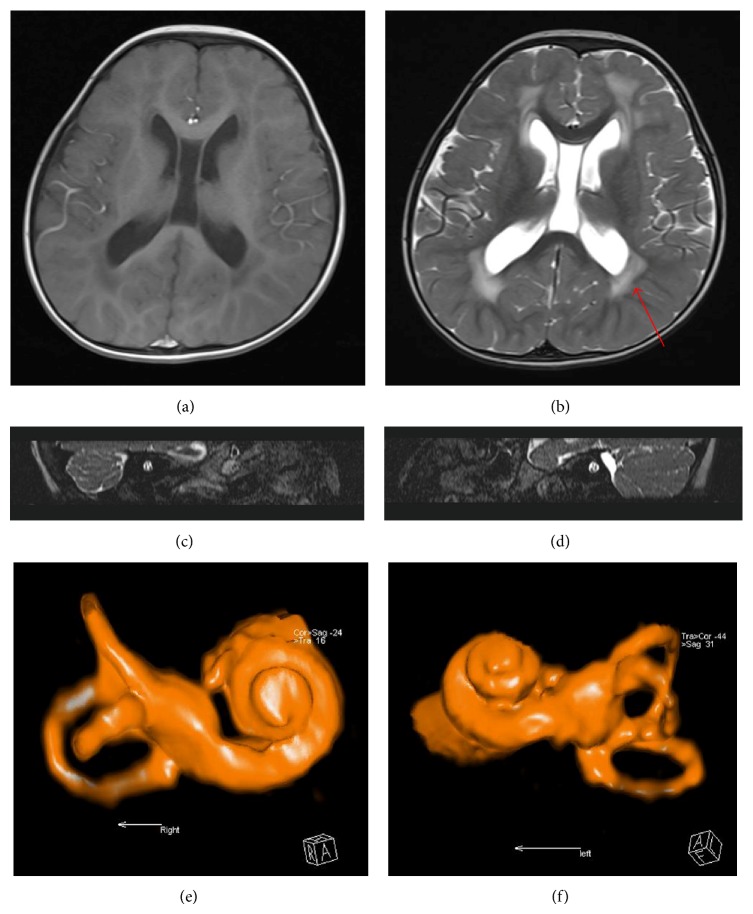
MR imaging of a representative patient with SNHL. (a, b) Axial T1 and T2 weighted imaging showed abnormal signal in the white matter in the bilateral periventricular area and enlargement of septum pellucidum. (c, d) Sagittal 3D-SPACE imaging showed the normal facial nerve, cochlear nerve, and vestibular nerves in the internal auditory canal. (e, f) Volume rendering imaging showed the normal structure of the inner ear. IT-MAIS score of this patient improved from 0 to 10 after CI.
